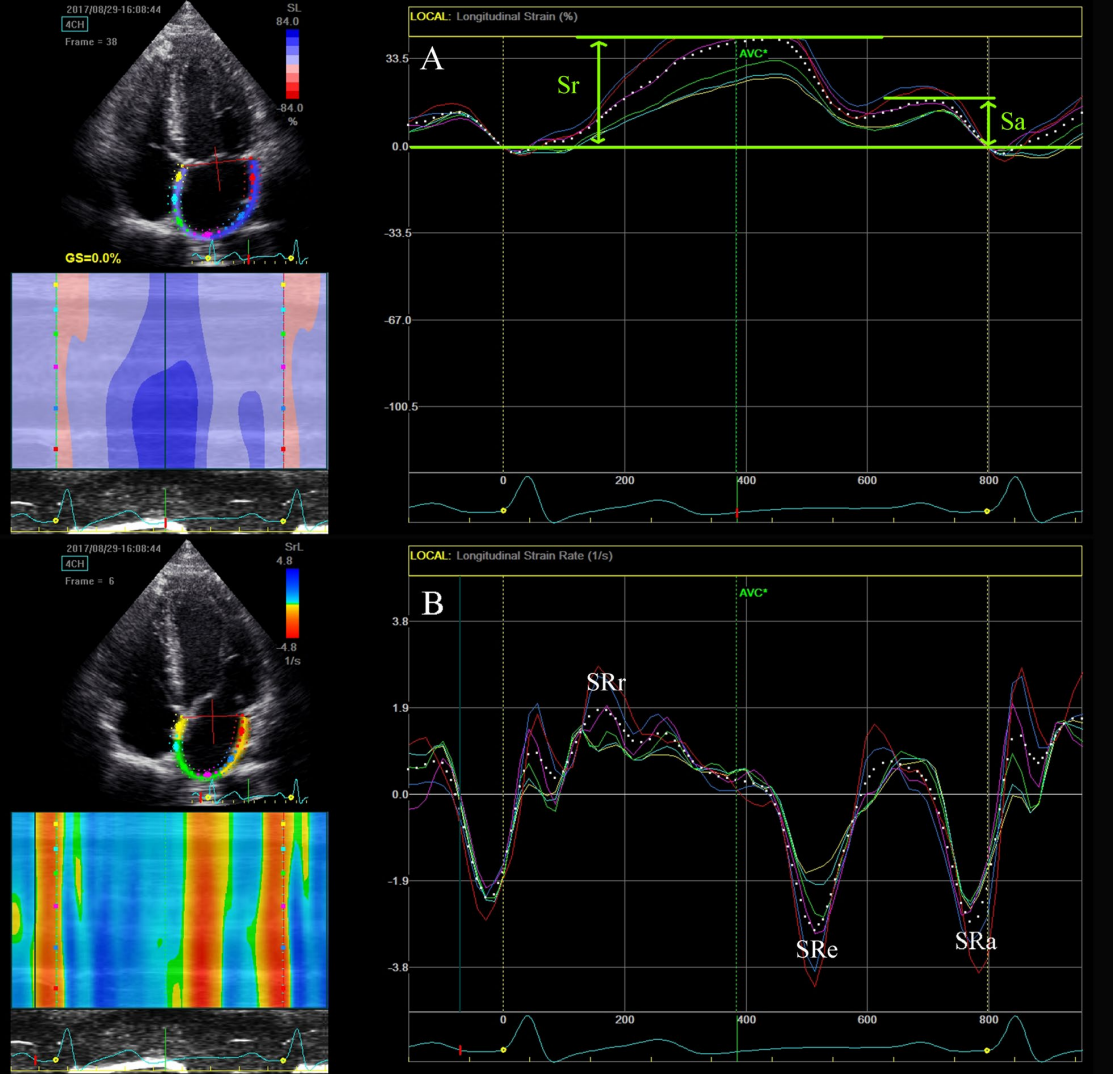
Speckle-tracking echocardiographic left atrial strain waves. A Left atrial longitudinal strain waves. Sr, LA total strain; Sa, LA active strain. B Left atrial longitudinal strain rate waves. SRr, LA total strain rate; SRe, LA passive strain rate; SRa, LA active strain rate
